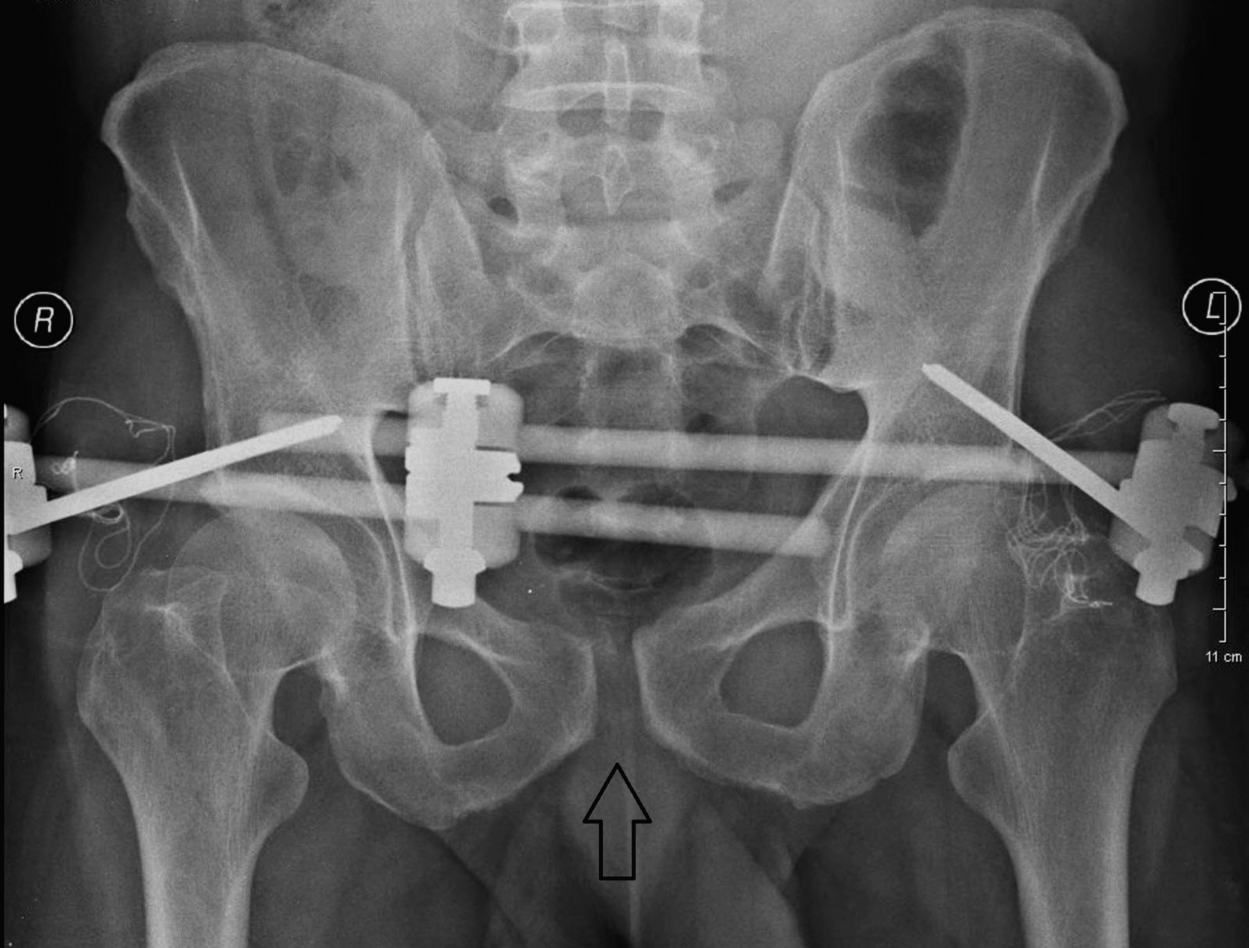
Anteroposterior X-ray after closed reduction and external fixation (First postoperative day).
